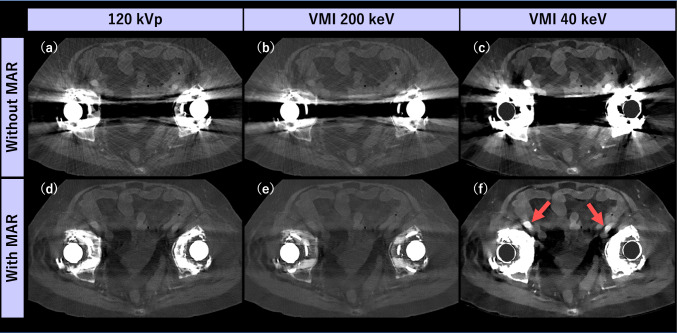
A 68-year-old woman with bilateral total hip replacement who underwent contrast-enhance CT using dual-layer detector scanner. Compared to conventional 120 kVp (a), VMI at 200 keV without MAR (b) shows limited artifact reduction and poor visualization of iodinated structures. In contrast, MAR-processed images (d–f) show significant reduction in metallic artifacts. The combination of 200 keV with MAR (e) is suitable for evaluating bone structures but offers no advantage for soft tissue assessment compared to 120 kVp with MAR (d). The best visualization of iodinated structure such as arteries (arrows) is obtained at VMI 40 keV with MAR (f)
